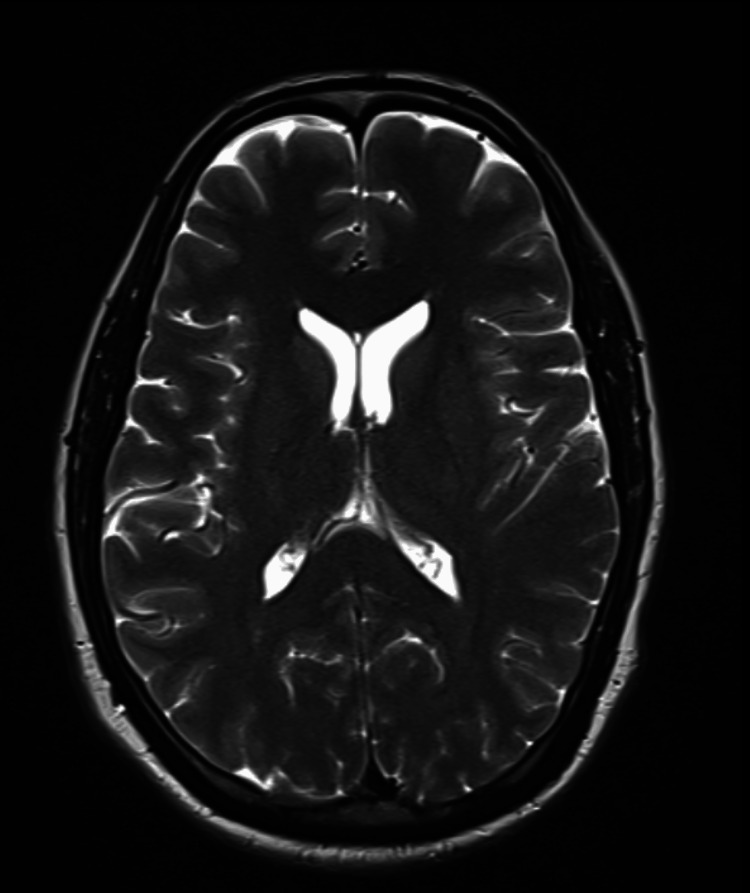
T2-weighted MRI of the brain parenchyma showing normal findings. MRI: magnetic resonance imaging.
